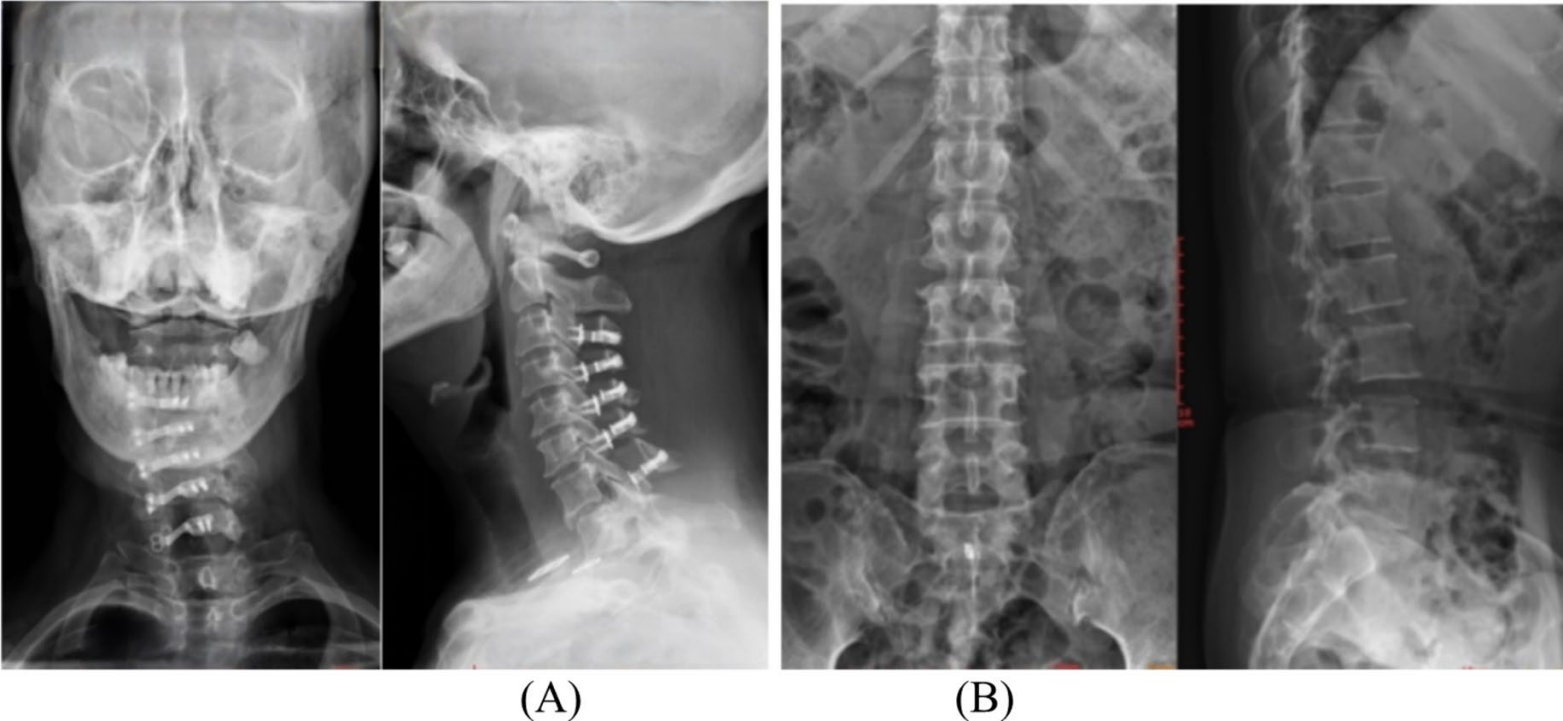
Anteroposterior X-ray and lateral X-rays (A) at the 5-month operation showed that the C7 lateral mass screws were missing and that the screws had migrated to the sacral canal (B)
